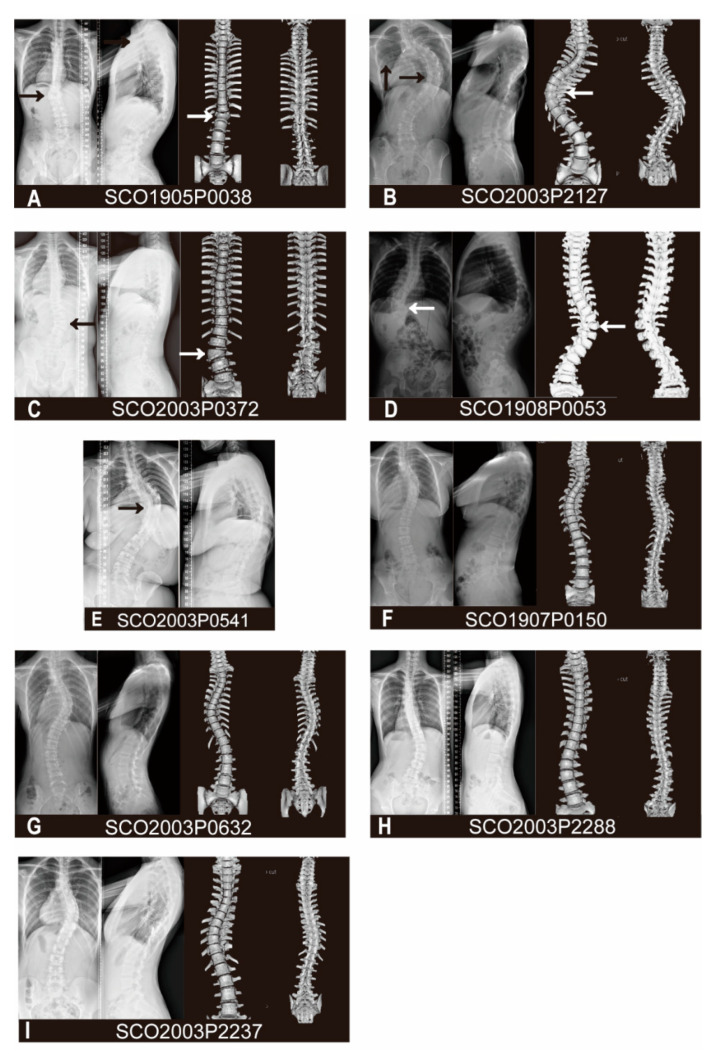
Antero-posterior, lateral spinal X-ray and the spinal three-dimensional CT reconstruction of patient SCO1905P0038 (A), SCO2003P2127 (B), SCO2003P0372 (C), SCO1908P0053 (D), SCO1907P0150 (F), SCO2003P0632 (G), SCO2003P2288 (H), SCO2003P2237 (I). Antero-posterior, lateral spinal X-ray of patient SCO2003P0541 (E). Arrowheads point to the vertebral or rib deformities.
